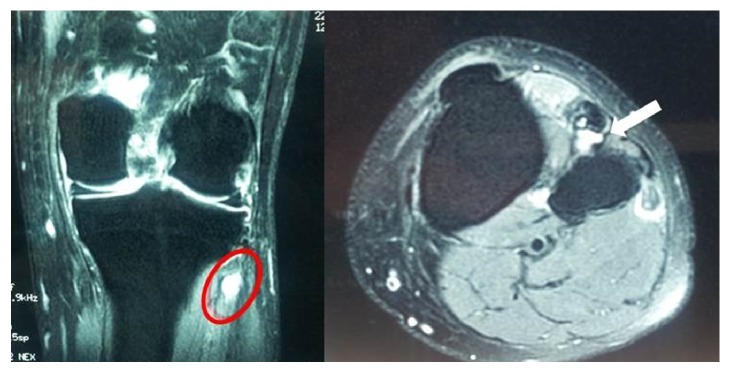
Left knee MRI demonstrated a lobulated, multilocular, and well-demarcated cystic-appearing mass medial to the fibular head (ganglion).
